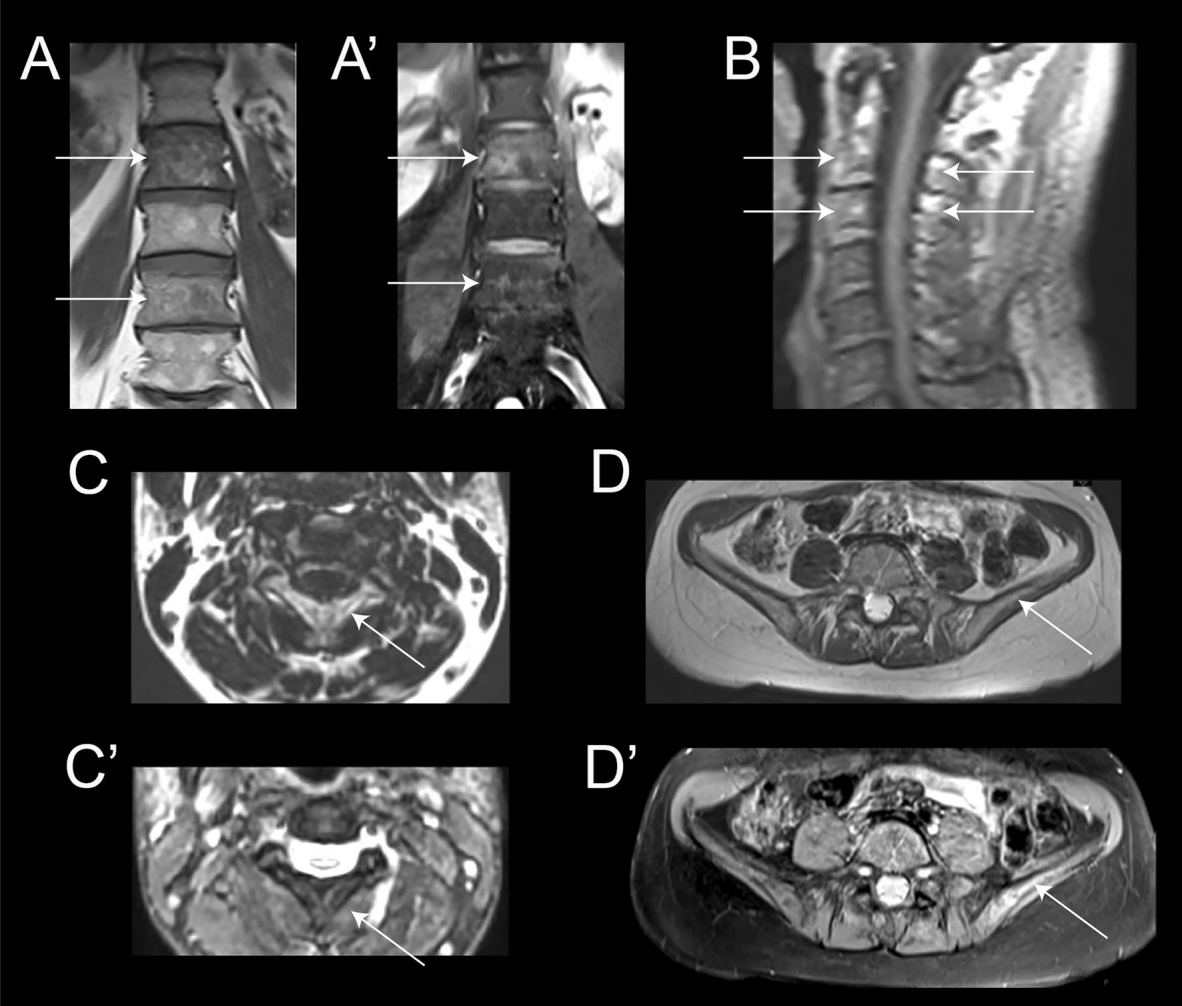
Examples of MRI finding in patients with Paget disease of the bone included in this study. Coronal views T1 (A) and T2 with fat saturation (A’)-weighted images of the lumbar spine showing heterogeneity of the signal of bone marrow at L2 and L4 levels compared to L1 and L3 (arrows). Coarsed trabeculae are more visible on T1 and edema of the vertebral body on T2 fat sat. Sagital (B) and axial (C and C’) T1-weighted and axial T2 with fat saturation views centered on the upper cervical spine. Signal heterogeneity, cortical thickening and bone enlargement of the entirety of C2 and C3 vertebrae is detectable (arrows). Axial T1 (D) and T2 (D’) with fat saturation-weighted images centered on the iliac crest. Bone changes are very subtle on T1 but bone edema and heterogeneity is more visible on T2 on the left side (arrow) especially in comparison with the right side
